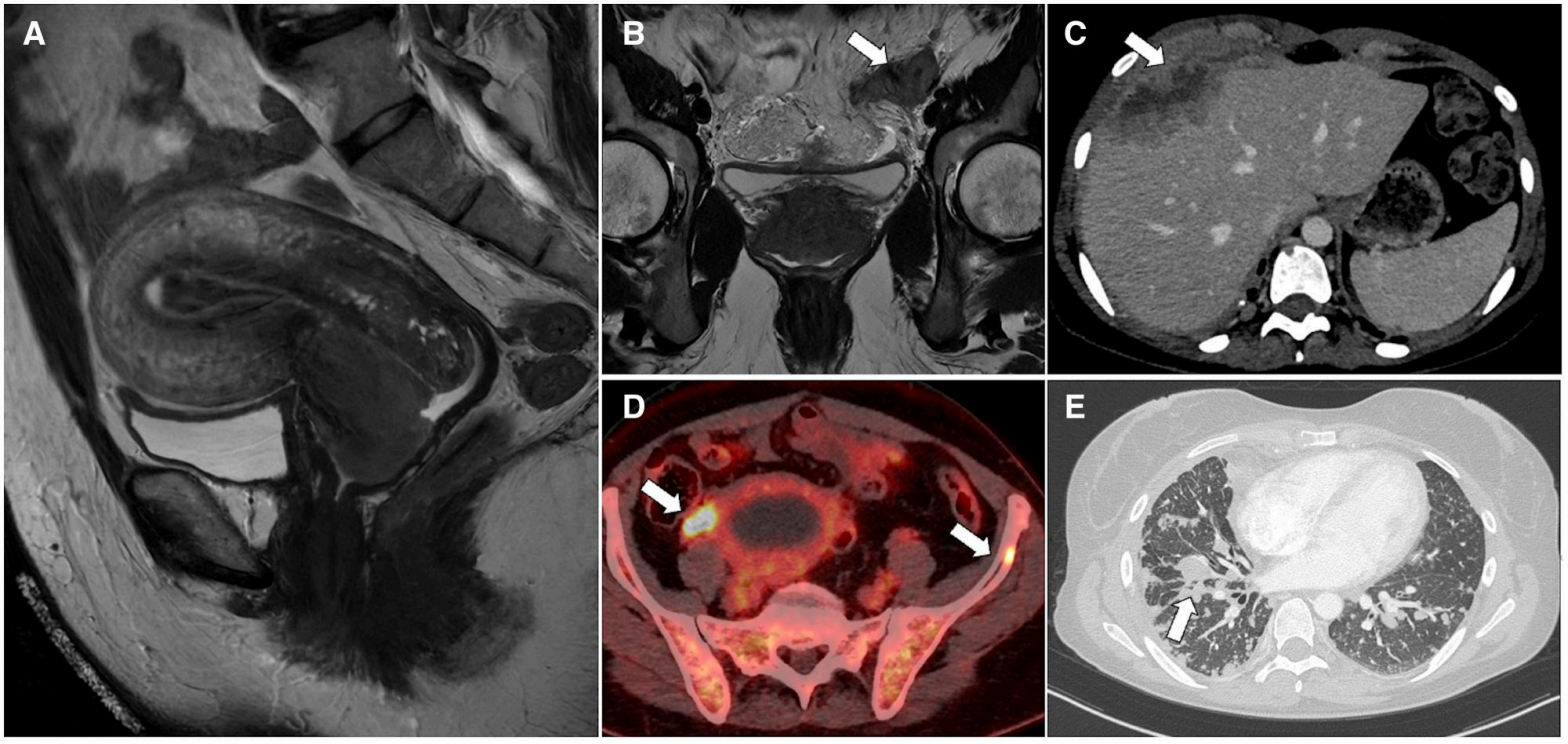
Neuroendocrine tumour of the cervix. Sagittal T2-WI (A) shows an intermediate-SI lesion in the anterior part of the cervix, which on MRI cannot be distinguished from a common squamous cell carcinoma. Due to the aggressive behaviour of neuroendocrine tumours, additional disease sites can be present (arrows), such as the peritoneum (B, C), ovaries (D), bones (D), and lungs (E). Abbreviations: SI = signal intensity; WI = weighted imaging.
